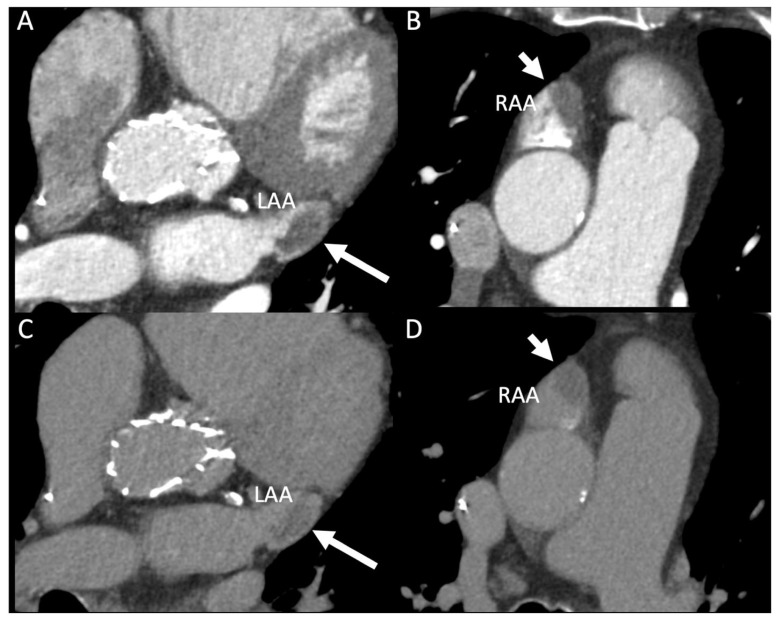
Contrast-enhanced cardiac computed tomography (CT) demonstrating simultaneous thrombi in the left and right atrial appendages. (A) Arterial-phase axial image showing a well-demarcated, low-attenuation filling defect within the left atrial appendage (long white arrow), compatible with thrombus. (B) Arterial-phase axial image demonstrating a similar filling defect within the right atrial appendage (short white arrow). (C) Delayed-phase image confirming persistence of the left atrial appendage filling defect without contrast enhancement (long white arrow), supporting the diagnosis of thrombus rather than slow-flow. (D) Delayed-phase image showing persistent non-enhancing material within the right atrial appendage (short white arrow), also consistent with thrombus.
